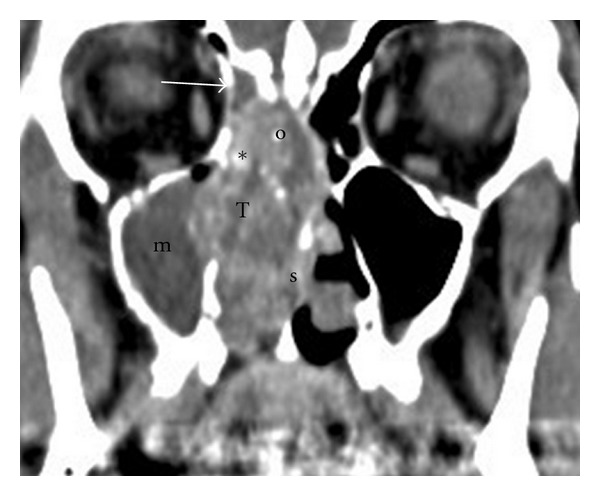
Initial lesion, CT scan: the tumor (T) is centered on the olfactory cleft (o), pushing the septum (s). The invasion of the middle turbinate and the presence of intratumoral calcifications (∗) could indicate the presence of chordoma. Ethmoidal attic (*→*) and maxillary sinus (m) are retentional.
